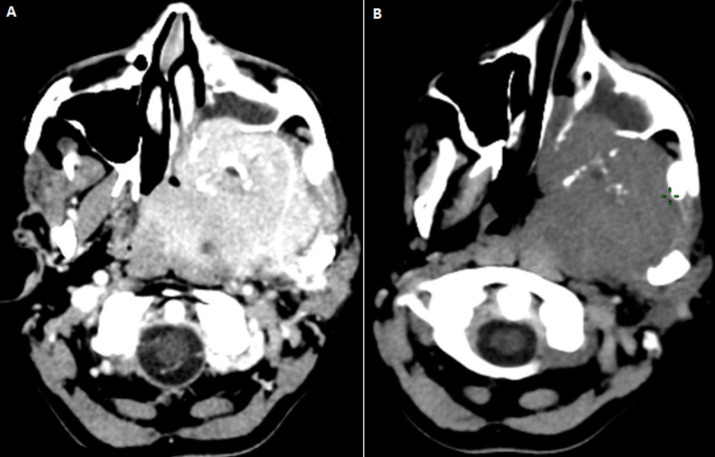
A) cervical CT scan in axial sections without and with injection showing the hypodense tumour of the cavum; B) enhancing intensely after injection of contrast agent. It occupies the left infra temporal fossa with invasion of the nasal fossa and the homolateral maxillary sinus
